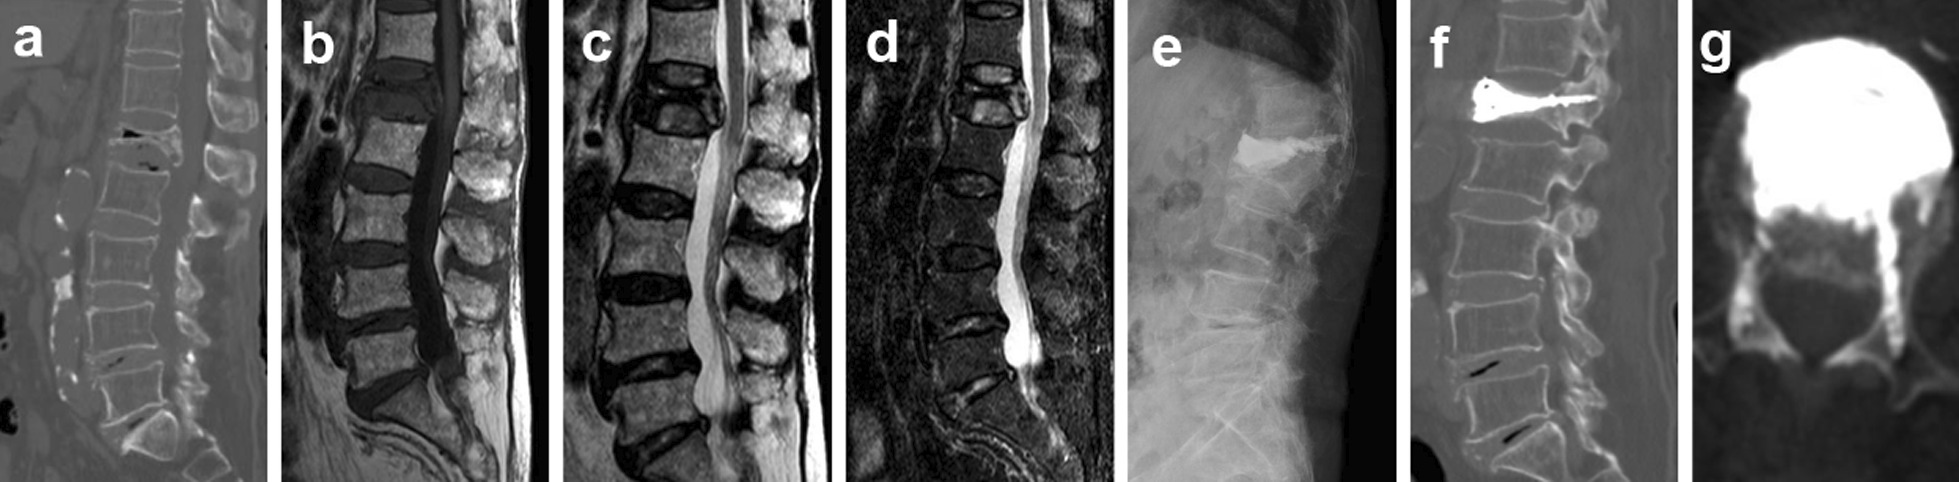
Treatment of Kümmell’s disease via percutaneous vertebroplasty combined with pediculoplasty (PVP-PP). A male aged 80 years complained of low back pain and discomfort for 5 months. Preoperative computed tomography (CT) revealed dehiscence of the anterior middle region of vertebra L1 and the formation of a vacuum fissure within the vertebra (a). Preoperative magnetic resonance imaging confirmed that the L1 vertebral body was a fresh fracture. The vacuum fissure in the vertebral body also evidenced a fluid signal (b-d). An X-ray taken on the first day after surgery revealed that the bone cement was evenly distributed in the vertebral body and the pedicle (e). A postoperative CT scan showed that the bone cement was evenly distributed in the vertebral body and bilateral pedicle without leakage into the vertebral canal (f, g)
